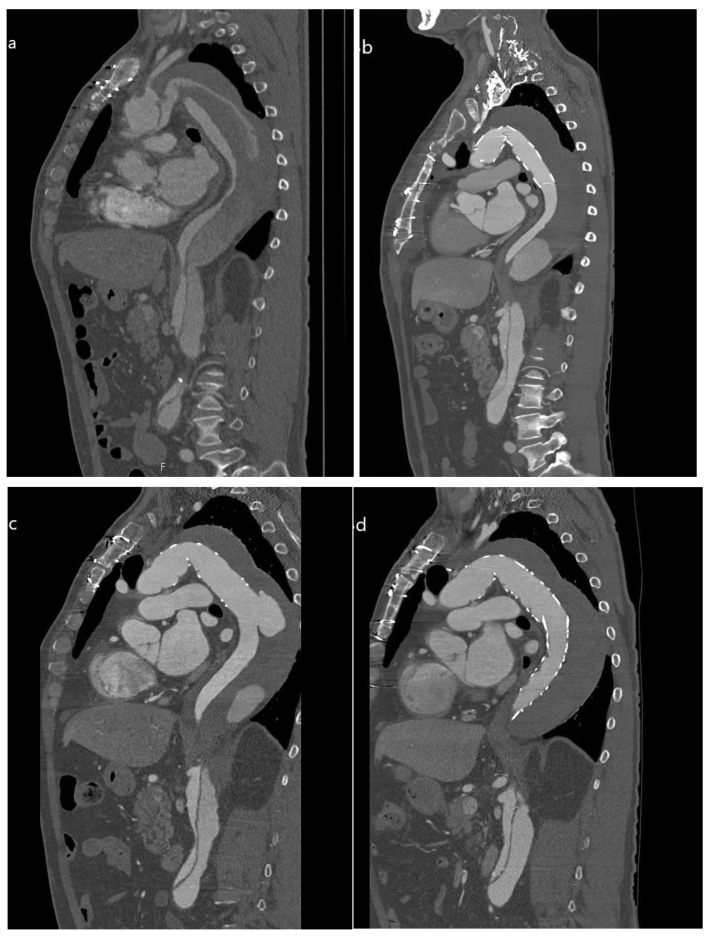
Computed tomography images of patient with acute type A aortic dissection treated with FET and subsequent TEVAR extension to address dSINE and aneurysmal growth. Patient with acute type A aortic dissection (a) was treated with FET. First postoperative imaging (b) showed satisfactory result, but follow-up imaging after 3 months (c) revealed dSINE with resulting aneurysmal growth requiring second-stage repair (d) with sufficient positive aortic remodeling.
